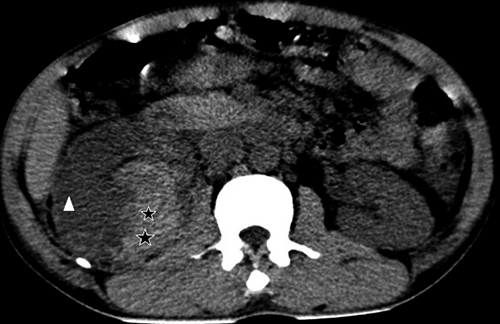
Ultrasound image of a subcapsular hematoma of the right kidney in axial section without injection of contrast medium. Spontaneous hyperdense structure under the right kidney capsule (black star) compressing the right kidney (white triangle).
